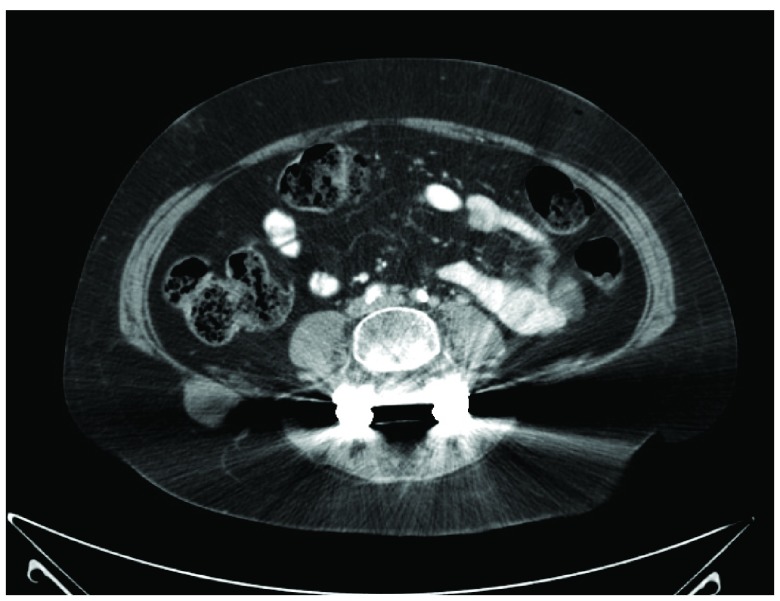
CT chest, abdomen and pelvis with contrast: A new 3 cm soft tissue mass is identified in the subcutaneous fat adjacent to the right iliac crest.
